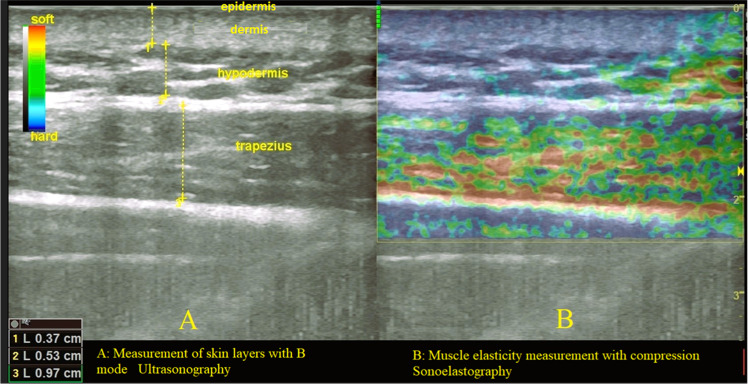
Measurement of skin-subcutaneous tissue thickness with B mode Ultrasonography and muscle elasticity measurement with Sonoelastography.
